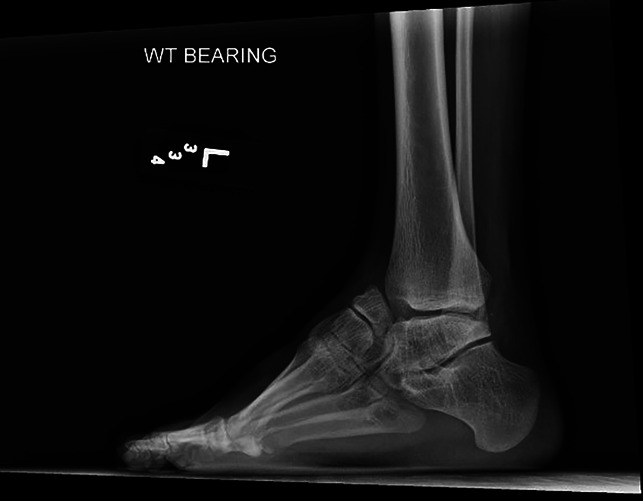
Lateral preoperative radiograph of the left foot and ankle.
